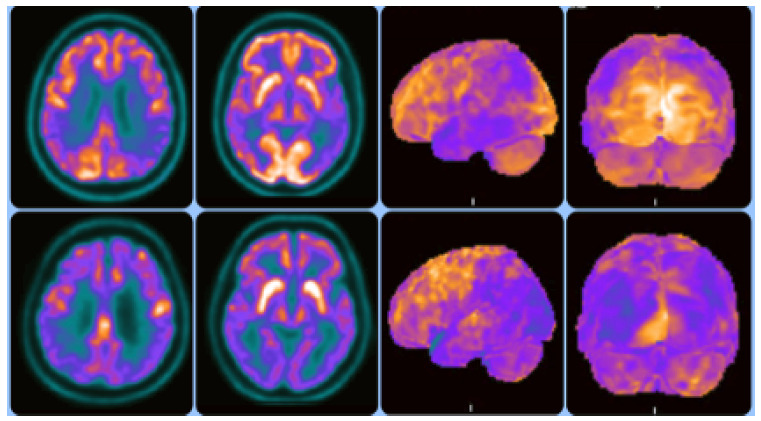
[^18^F]FDG-PET images in Alzheimer’s disease (AD) and Dementia with Lewy Bodies (DLB). Upper row - Images on the left with standard axial view and images on the right with [^18^F]FDG-PET 3D-stereotactic surface projection (3D-SSP, Cortex ID Suite software, GE Healthcare):occipital lobe metabolism is typically preserved in AD. Hypometabolism in AD usually occurs in bilateral temporoparietal regions. Lower row - Images on the left with standard axial view and images on the right with [^18^F]FDG-PET 3D-stereotactic surface projection (3D-SSP, Cortex ID Suite software, GE Healthcare): DLB shows occipital hypometabolism. Relative preservation of the posterior cingulate region, representing the “cingulate island sign”. (Reproduced with permission from Dr. Artur Coutinho, Nuclear Medicine Center - Inrad - HC-FMUSP).
